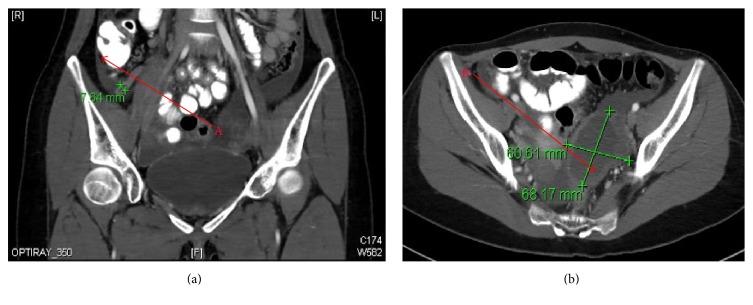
CT scan. A: inflamed appendix; B: left adnexal mass.
